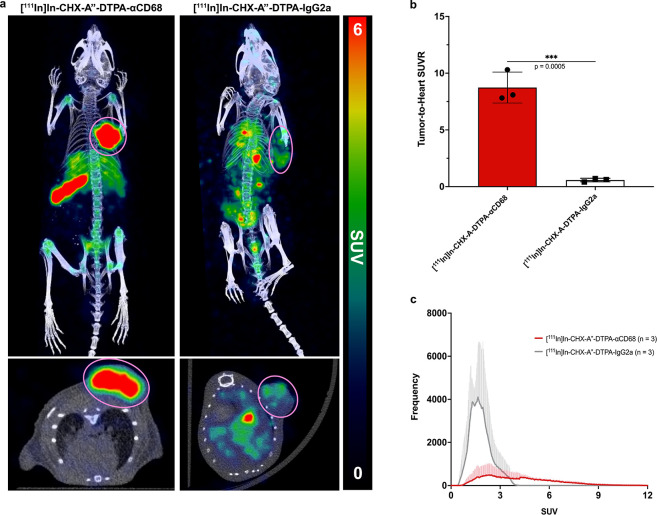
ImmunoSPECT with [^111^In]­In-CHX-A″-DTPA-αCD68
